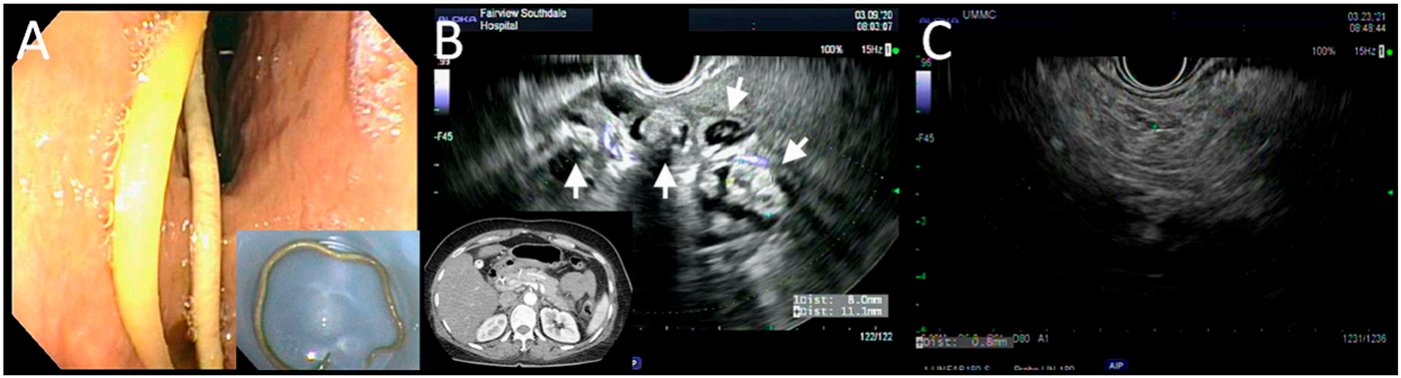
(A) Endoscopic visualization of the *Ascaris* worm in the antrum of the stomach. (B) Endosonographic images of cystic structures (arrows) adjacent to the tail of the pancreas. (C) Repeat endoscopic ultrasound showing resolution of findings after anthelmintic treatment. This figure appears in color at ajtmh.org.
